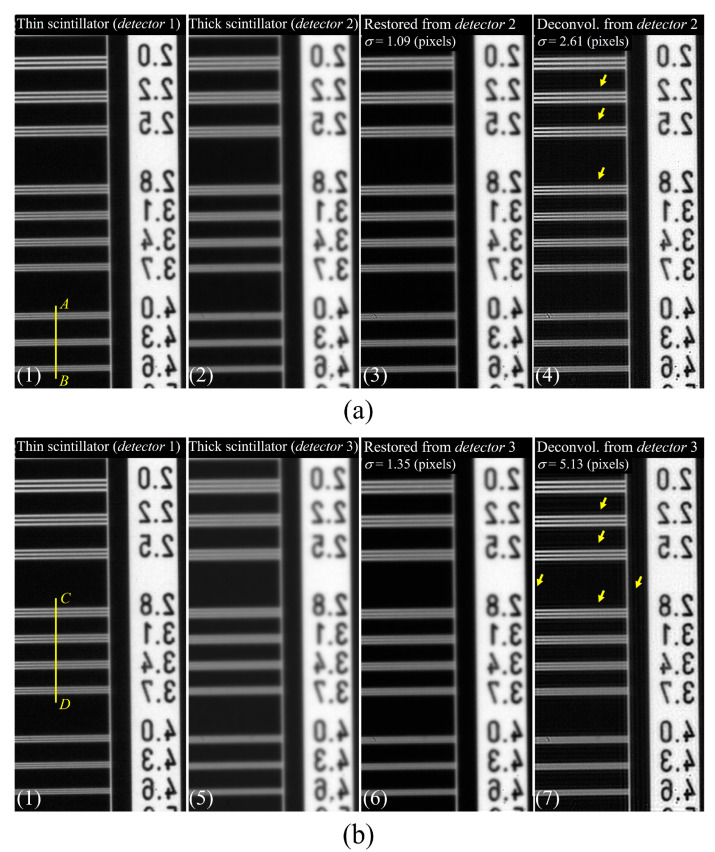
Experimental results of the radiographic image with a thin scintillator for detector 1, the degraded image with a thick scintillator, the proposed image derived from the thick scintillator image, and the deconvolution images utilizing the measured PSF to evaluate system performance. Here, (a) represents the outcomes between detectors 1 and 2, and (b) similarly depicts the outcomes between detectors 1 and 3.
